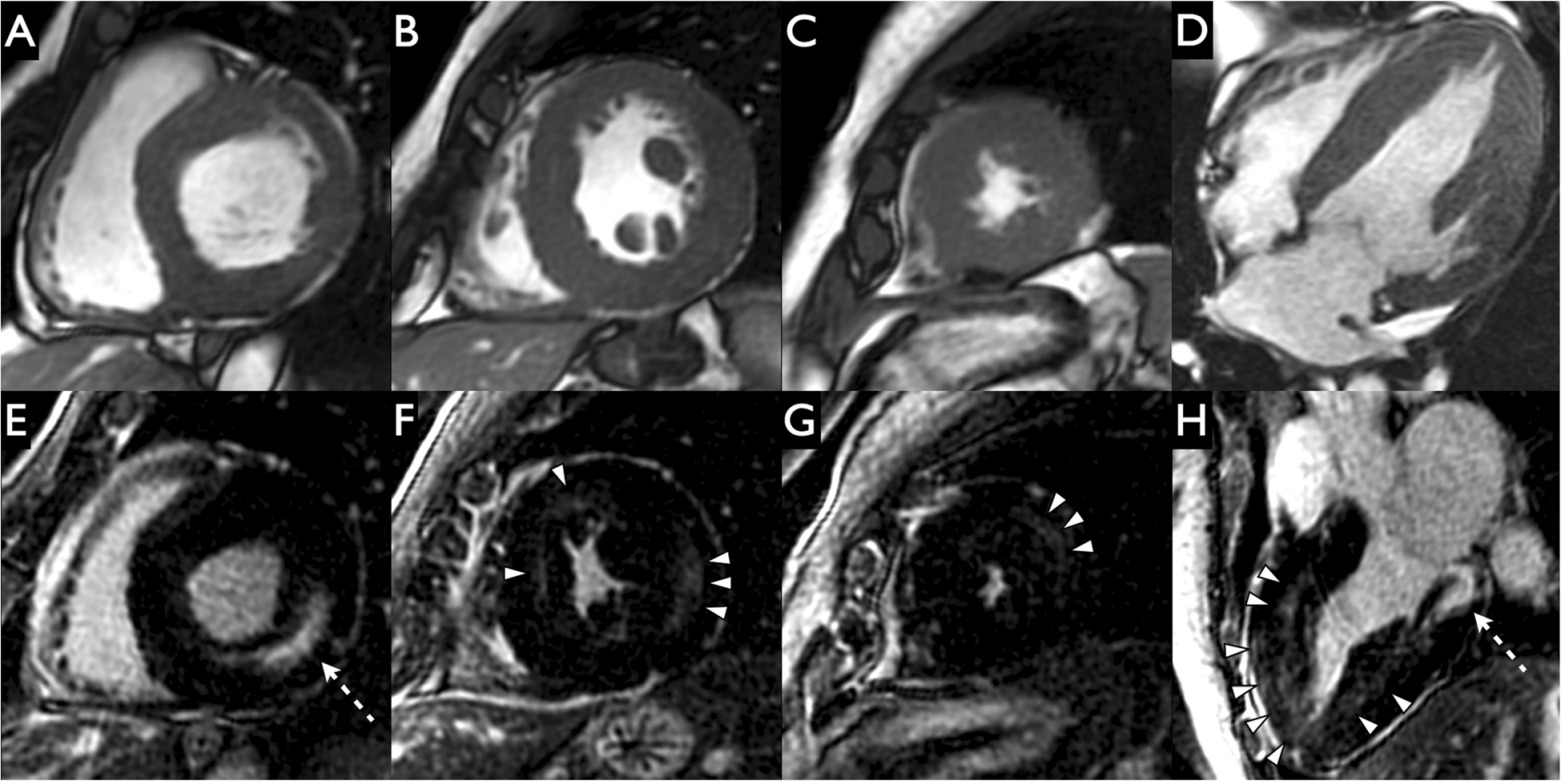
Concentric hypertrophy in Anderson-Fabry Disease (AFD). Short-axis (a-c) and 4-chamber (d) cine steady state free precession and short-axis (e-g) and 3-chamber (h) late gadolinium enhancement images in a patient with Anderson-Fabry disease who presented who presented initially with a wide complex tachycardia. Cardiovascular magnetic resonance imaging revealed typical concentric hypertrophy and high intensity inferolateral midwall scar (dashed white arrows in images e and h. There was also some intermediate intensity mid and apical left ventricular mid-myocardial scar (white arrowheads in images f-h. There was more scar in the basal LV than the mid and apical LV (Basal LV scar 15 %; Mid LV scar 8 %; Apical LV scar 11 %)
